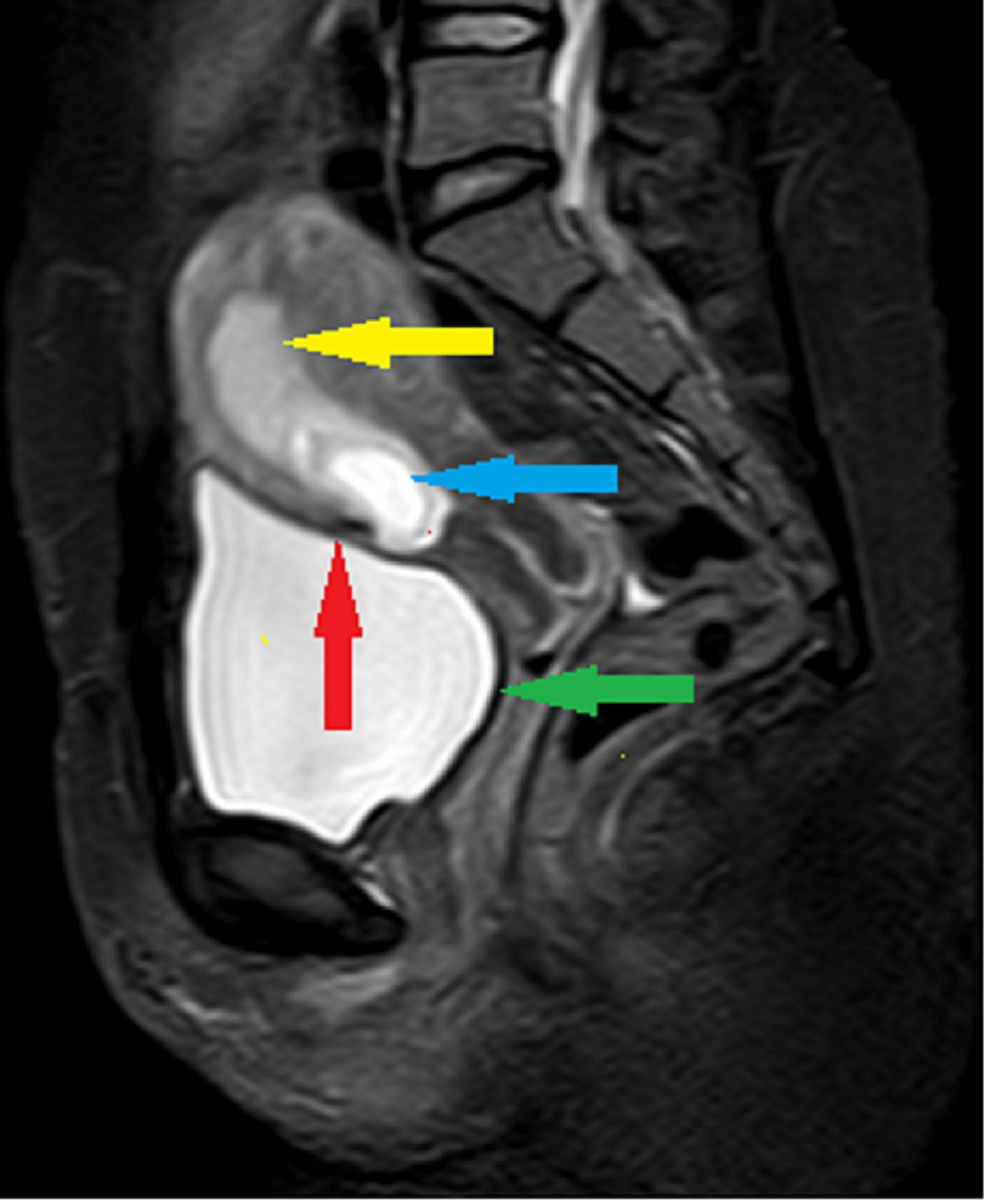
MRI pelvis T2W FAT-SAT sagittal image showing empty gestational sac located in lower uterine segment partially attached to the previous cesarean (blue arrow) within the endometrium (yellow arrow). The myometrium between the sac and bladder wall appears very thin (red arrow). Bladder wall appears intact and smooth (green arrow). T2W FAT-SAT, T2-weighted fat-saturation.
